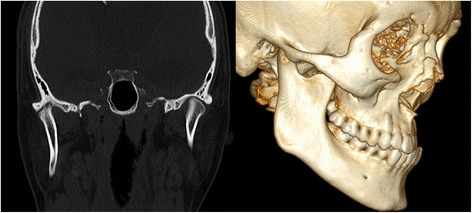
CT scan images of TMJ. Patients with history of trauma shows ankylosed TMJ on CT scan image, and 3D reconstruction demonstrates the overall shape of TMJ.
